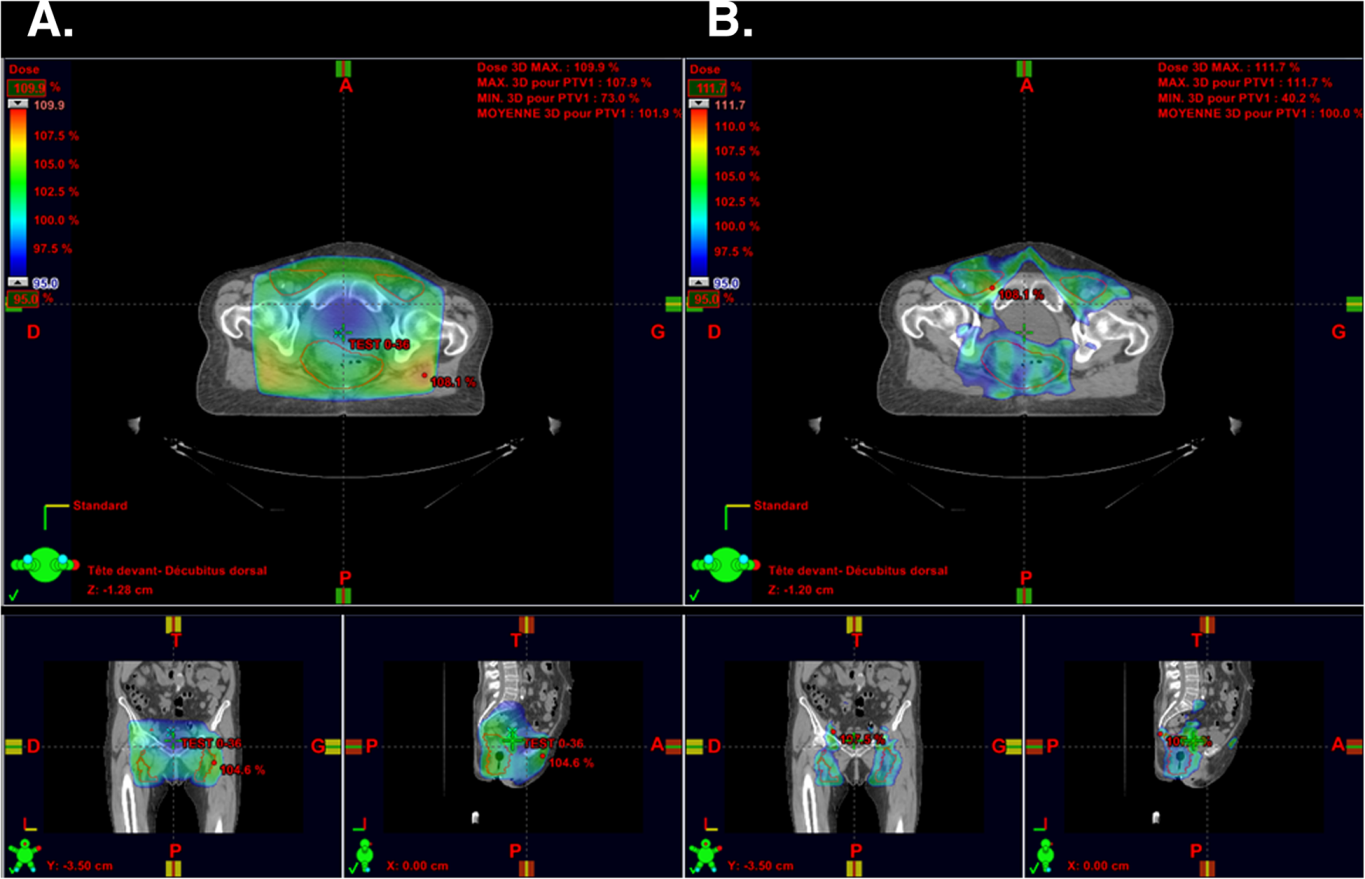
Comparison of dose distribution on planning CT with 3D-CRT and IMRT for a same patient with a locally advanced T3N+ anal cancer. Legend: The isodose distribution shows up in colorwash with the 95% isodose line selected Left column (A.) axial CT slice using 3D-CRT technique with small coronal and sagittal views above; Right column (B.) axial CT slice using IMRT technique. with small coronal and sagittal views above.
